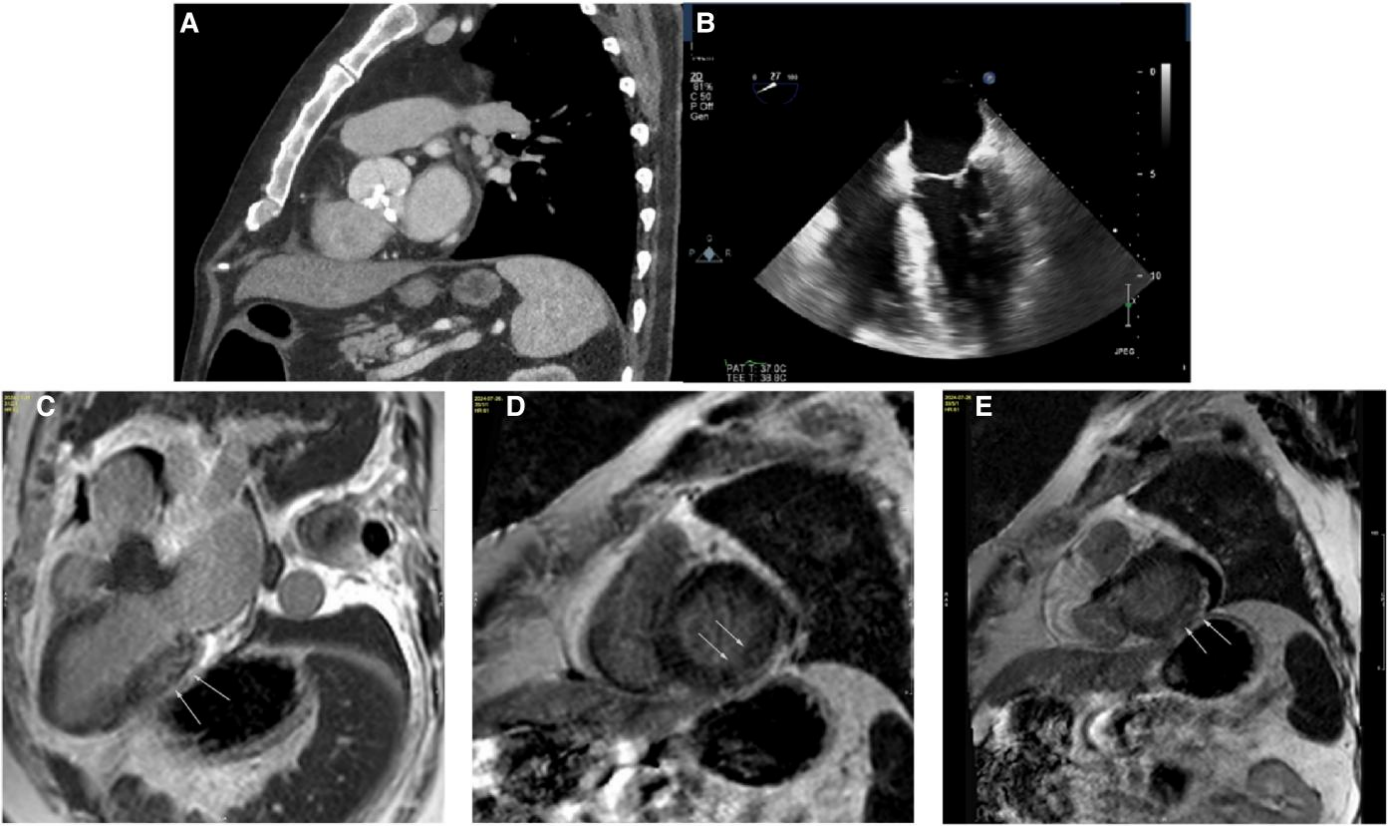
Cardiac imaging. Upper Panel: (*A*) Pre-operative chest computed tomography scan in the sagittal plane demonstrating a heavily calcified aortic valve (left). (*B*) transoesophageal echocardiography demonstrating increased concentric wall thickness with preserved biventricular systolic function (right). Lower Panel: Cardiac magnetic resonance imaging (*C*) Long axis 3 chamber view, white arrows showing patchy mid wall enhancement (non-ischemic fibrosis). (*D*) and (*E*) Short axis view, white arrows highlighting combination of patchy mid wall and subepicardial enhancement on the basal inferolateral segment of the left ventricle wall.
